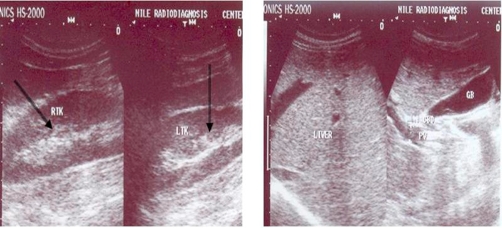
Pre-operative ultrasonographic figures showing normal right and left kidneys as well as normal liver and biliary system.
